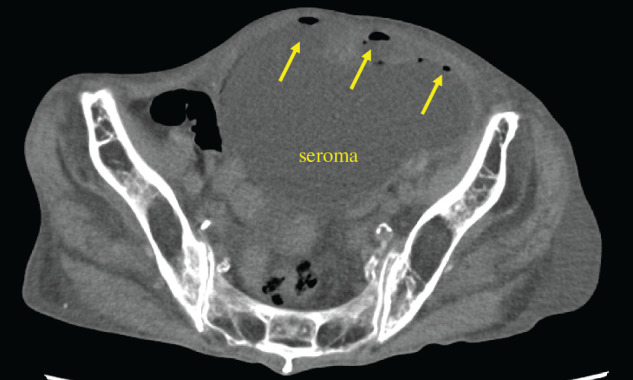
CT on POD 61 demonstrating the previously identified seroma with newly developed intralesional air (arrows), which was absent on prior imaging.
